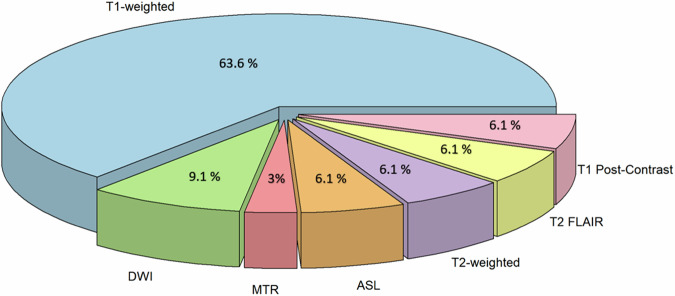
Distribution of the MRI input modalities/sequences used in synthetic PET studies. ASL, Arterial spin labeling; DWI, Diffusion-weighted imaging; FLAIR, Fluid-attenuated inversion recovery; MTR, Magnetization transfer ratio
